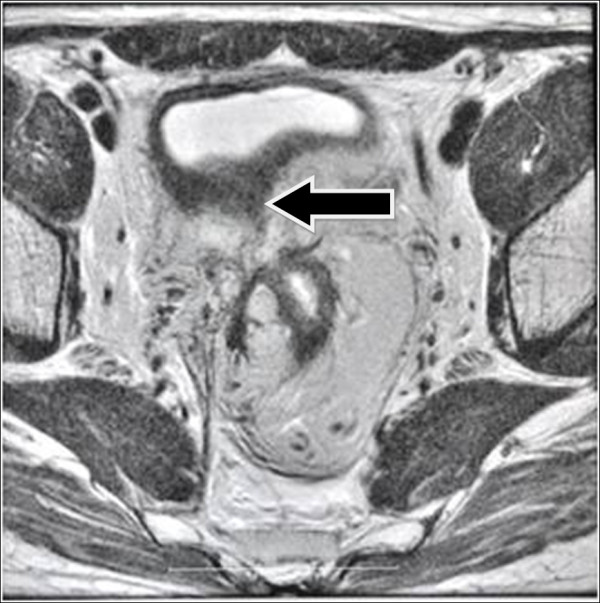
Magnetic resonance image of rectal cancer after neoadjuvant radiotherapy. The arrow indicates closure of rectovesical fistula.
